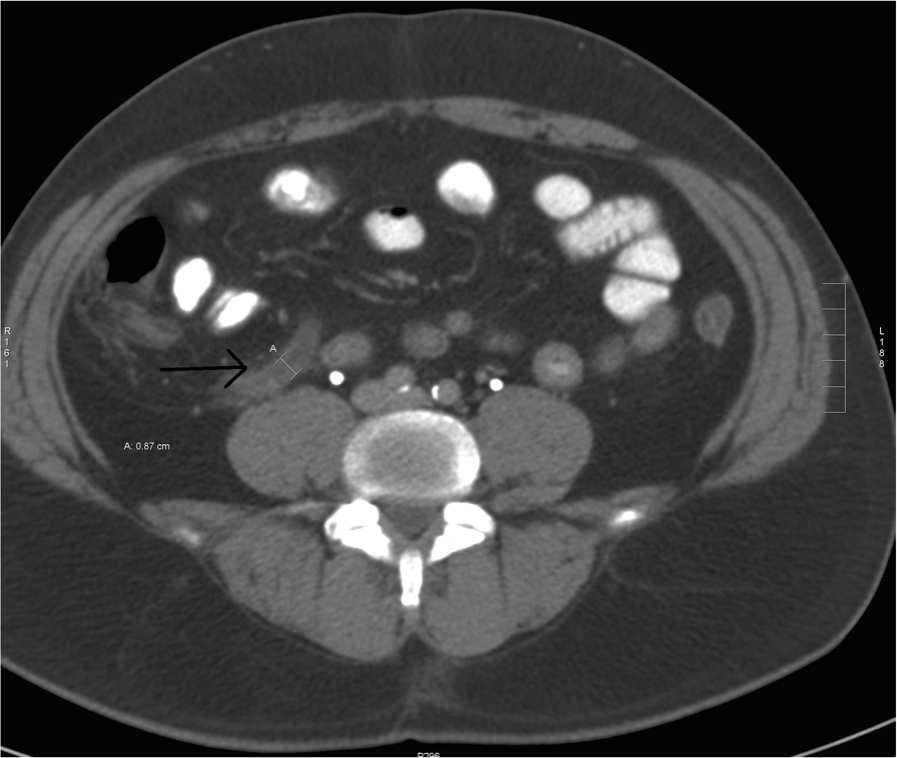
CT scan showing an inflamed appendix.
